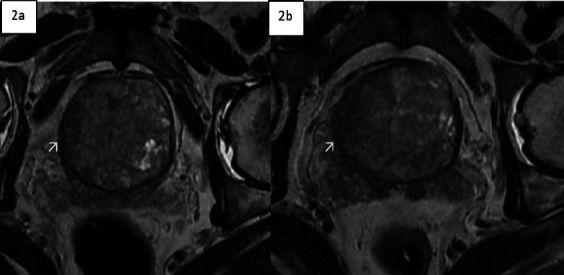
T2W axial MR images at different levels showing focal T2 hypo-intense lesion located in the right lateral side of an enlarged transitional zone of the prostate (2a shown with an arrow) and extension to the peripheral zone and areas of extra-prostatic extension (2b shown by the arrow
